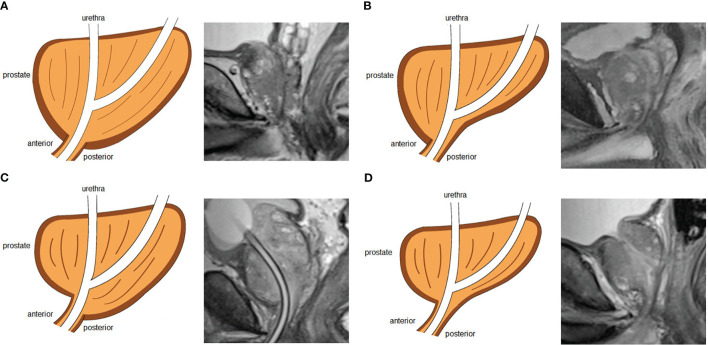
Four types of prostate apical shapes on mid-sagittal magnetic resonance imaging scan: (A) apical type 1: apex covering both the anterior and posterior sides of the membranous urethra; (B) apical type 2: apex only covering the anterior aspect of the membranous urethra; (C) apical type 3: apex only covering the posterior side of the membranous urethra; (D) apical type 4: apex not covering the membranous urethra.
